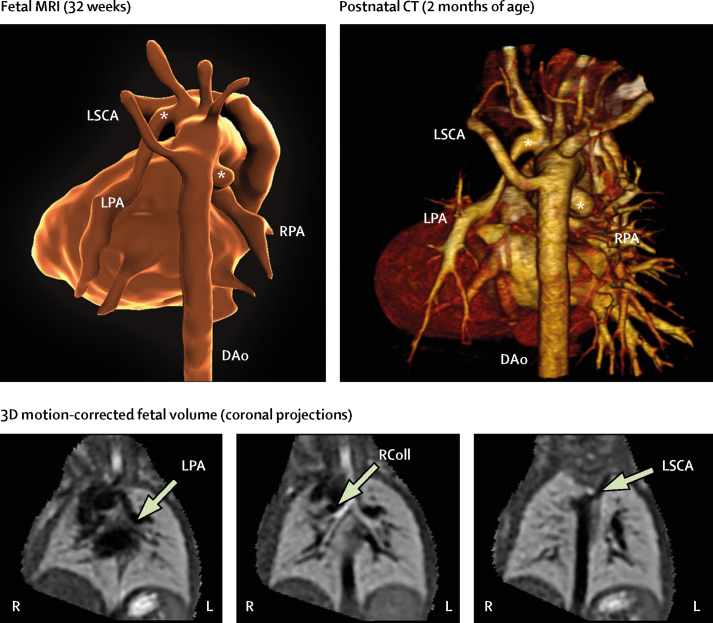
Segmentation of a fetal heart from 3D data at 32 weeks in a fetus with pulmonary atresia and ventricular septal defect, right aortic arch, and disconnected pulmonary arteries Fetal MRI showed two large collateral arteries (asterisks): the left pulmonary artery (LPA) was supplied by a large collateral vessel originating close to the origin of the left common carotid artery, with the right pulmonary artery (RPA) supplied by a tortuous collateral or arterial duct from the underside of the aortic arch. The left subclavian artery (LSCA) was also noted to be aberrant. All findings were confirmed with postnatal CT at 2 months (right panel). The bottom panel shows three coronal planes of the original 3D motion-corrected fetal data. These images were used for pre-birth surgical planning and parental counselling. DAo=descending aorta. RColl=right collateral.
